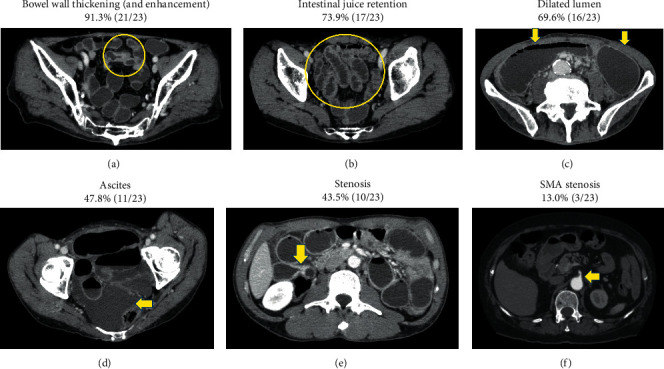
Positive computed tomography findings in patients with ischemic enteritis.
